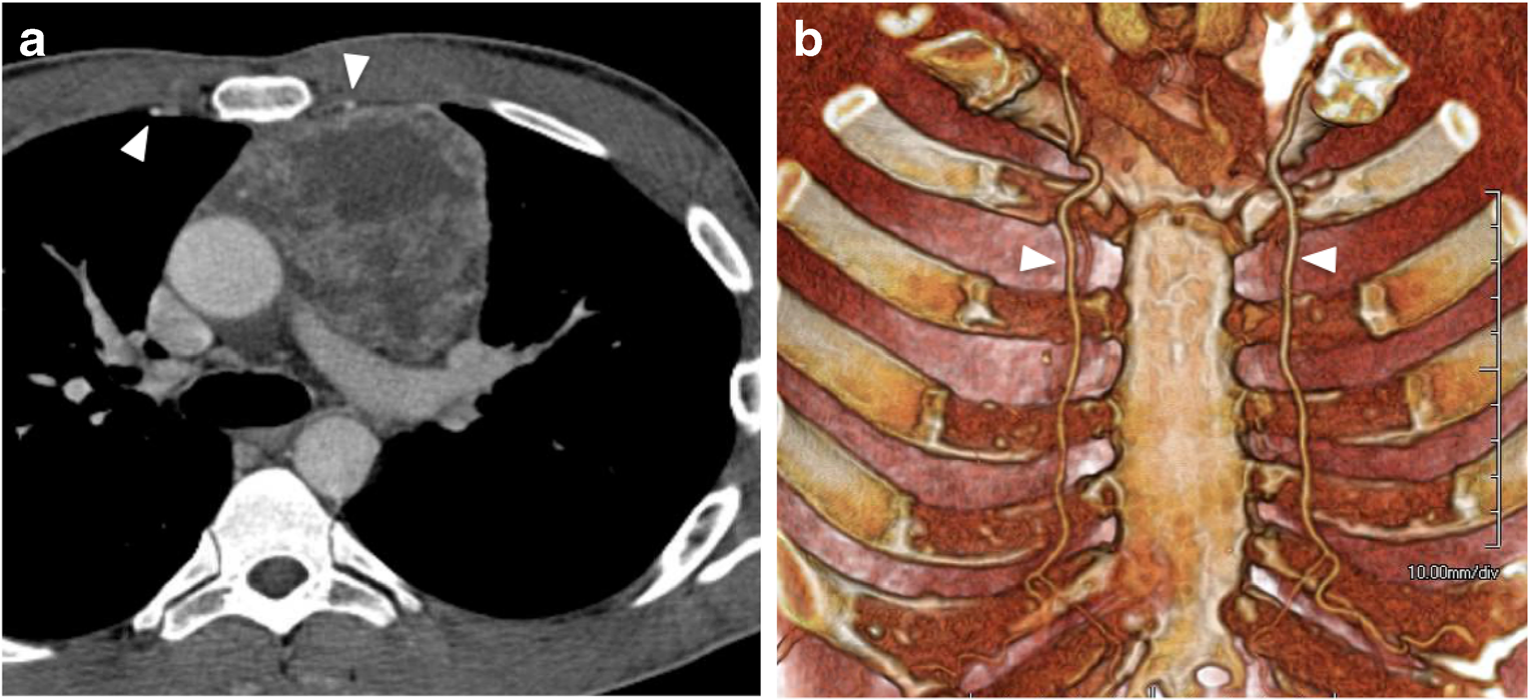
(a) Axial CT image and (b) 3D reconstruction showing internal mammary arteries (arrows), which must be avoided during the procedure
